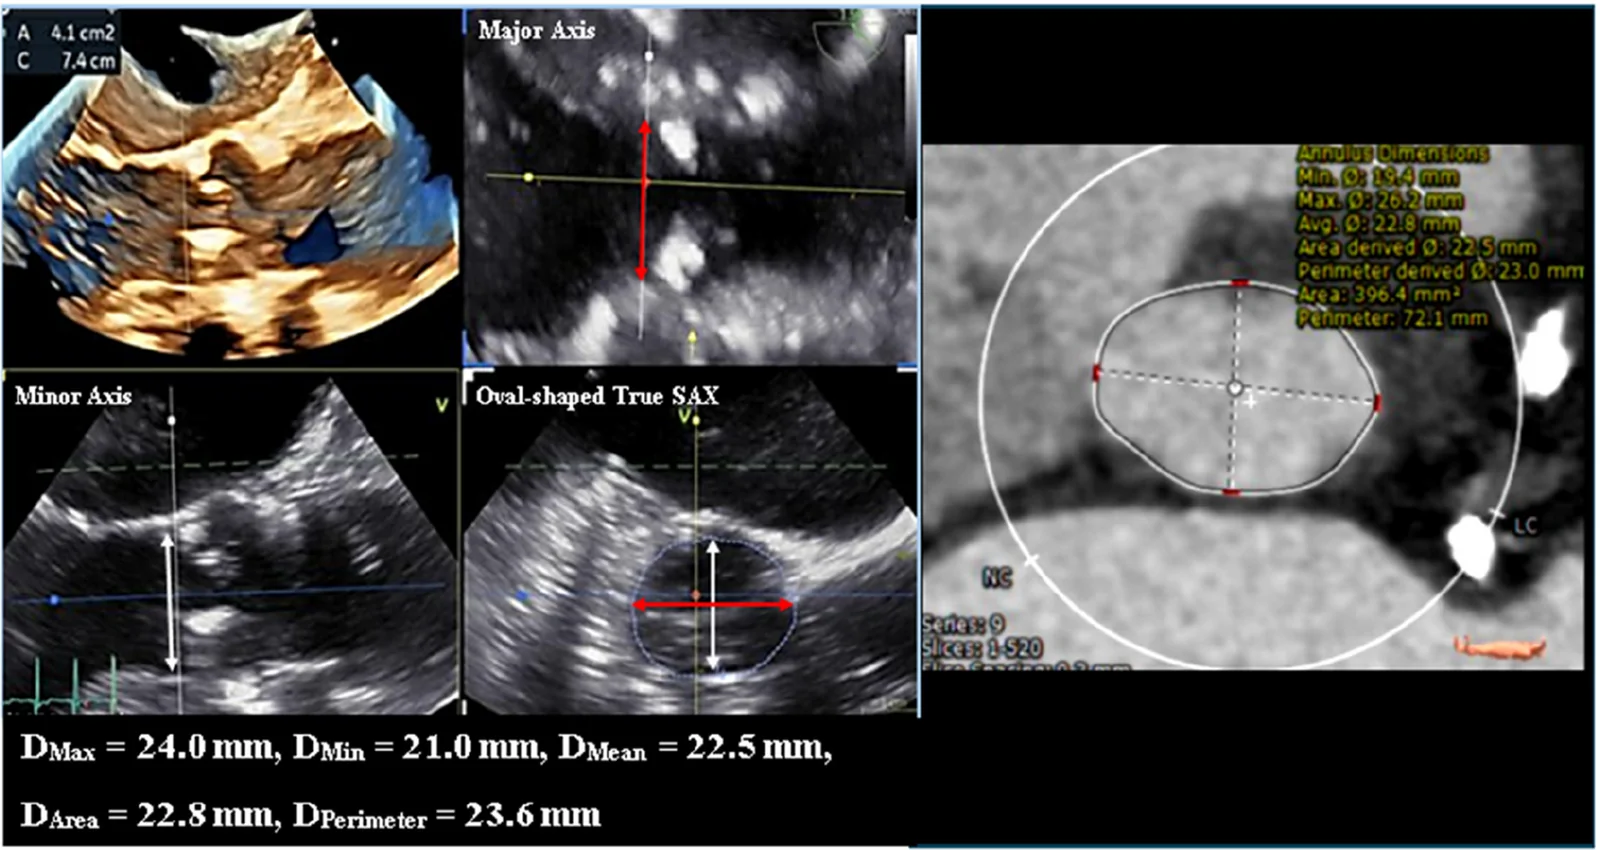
The 3D multiplanar imaging for the aortic annulus on transesophageal echocardiography (TEE). Left panel shows annular dimension obtained on 3D TEE via direct planimetry method using user-defined multiplanar approach, whereby the annular plane is a virtual plane at the level of the lowest attachment site of the three aortic cusps. As the aortic annulus is oval in shape, the long-axis plane diameter (from 2D TEE) typical represents its minor dimension (white arrow), while its maximal dimension is derived from the coronal plane obtained using multiplanar reconstruction of the acquired 3D volume dataset (red arrow). From 3D TEE, the annular area and perimeter were 410 mm^2^ and 74 mm, respectively. The right panel shows a computed tomography–derived aortic annular dimensions in the same patient. SAX, short axis
